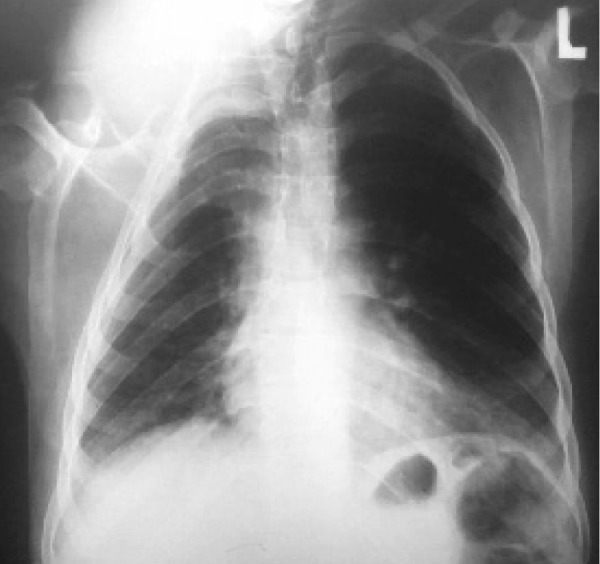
A fifteen-year-old boy with swelling of the right lower neck. PA chest X-ray
